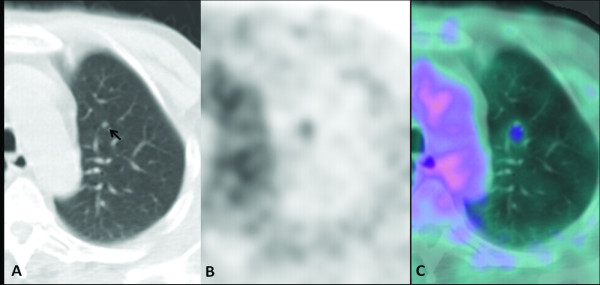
The smallest nodule. ^18^ F-FDG PET-CT: CT (a), PET (b), and fused (c) axial images. A focal ^18^ F-FDG uptake was evident in a very small nodule (black arrow) located in the upper left lobe.
